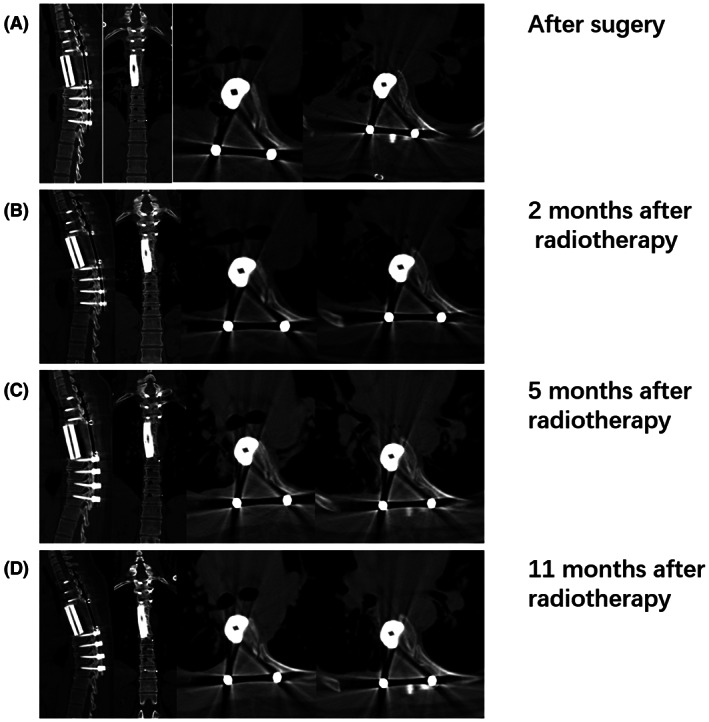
A typical patient who undergoes 3D‐printed vertebral implantation combined with high‐dose radiotherapy had CT images of 3D‐printed part at different time points.
